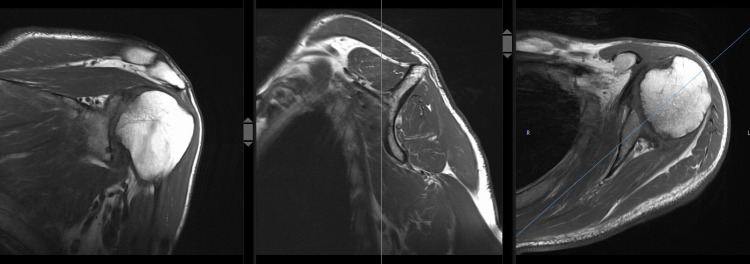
Coronal (left), sagittal (middle), and axial (right) T1-weighted turbo spin-echo MRI of the left shoulder with post-infectious arthritis, partial humeral head collapse but intact rotator cuff
